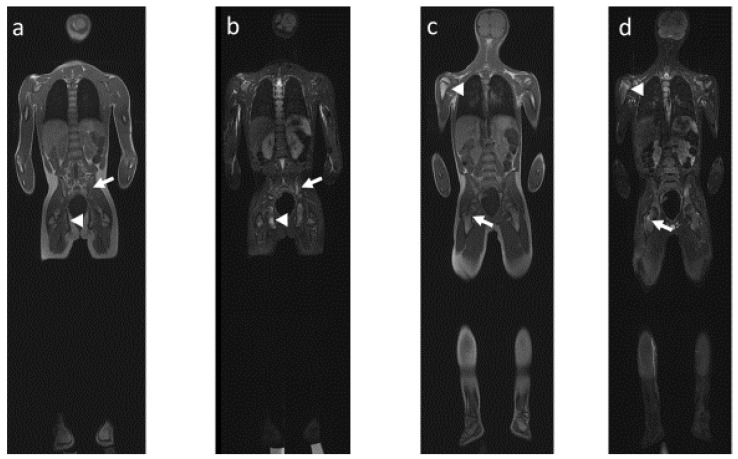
Whole body MRI, 12-year-old boy with confirmed CRMO. Coronal plane in (a) T1-weighted sequence and (b) STIR-weighted sequence showing bone marrow edema in the left iliac wing (arrow) and the right pubic ramus (arrowhead). Coronal plane (same exam) in (c) T1-weighted sequence and (d) STIR-weighted sequence showing bone marrow edema in the metaphysis of the right upper femur (arrow) and the metaphysis of the right upper humerus (arrowhead).
